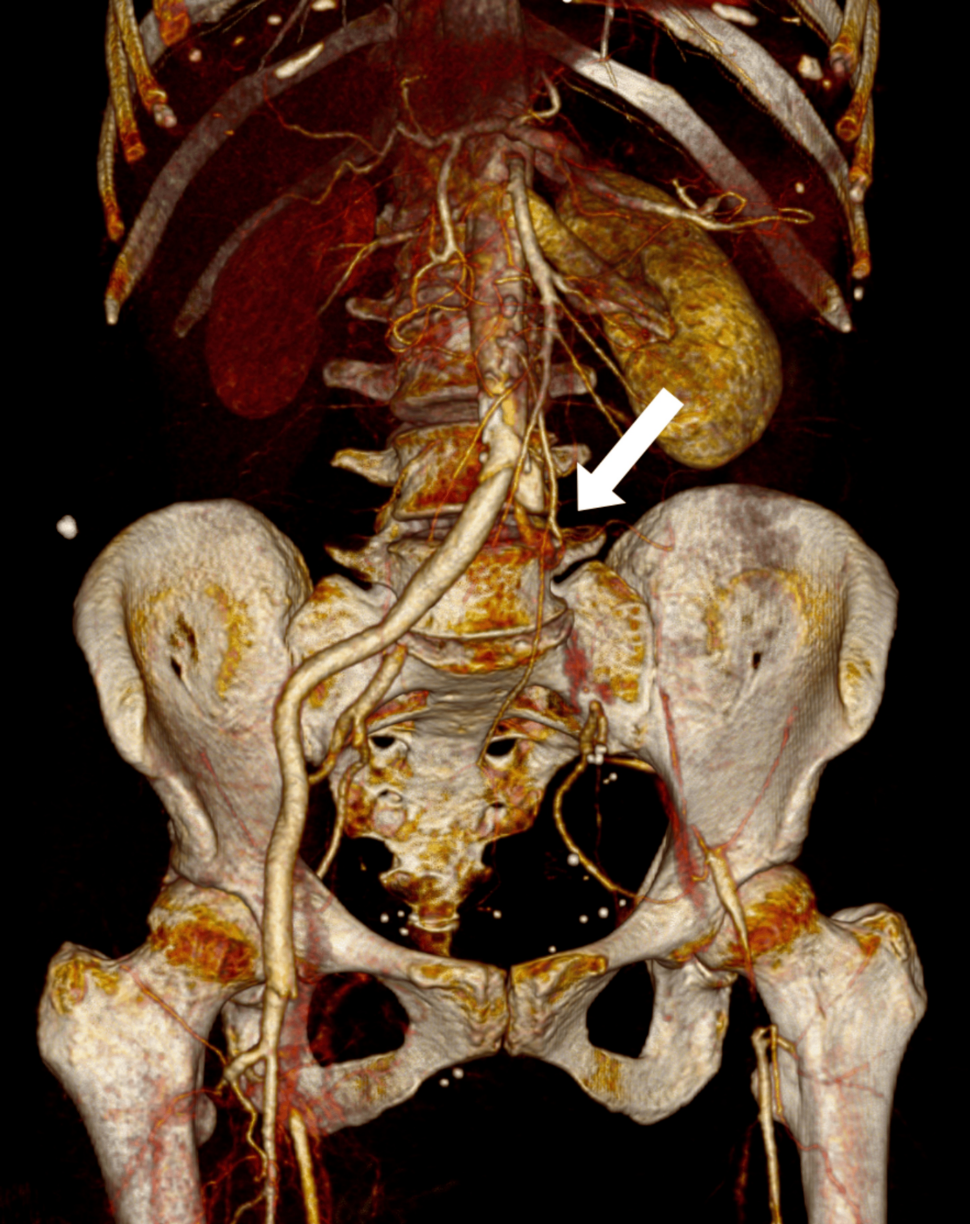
3D CT Angiographic Reconstruction The white arrow indicates a critical absence of flow in the left common iliac artery 3D, three-dimensional; CT, computed tomography
